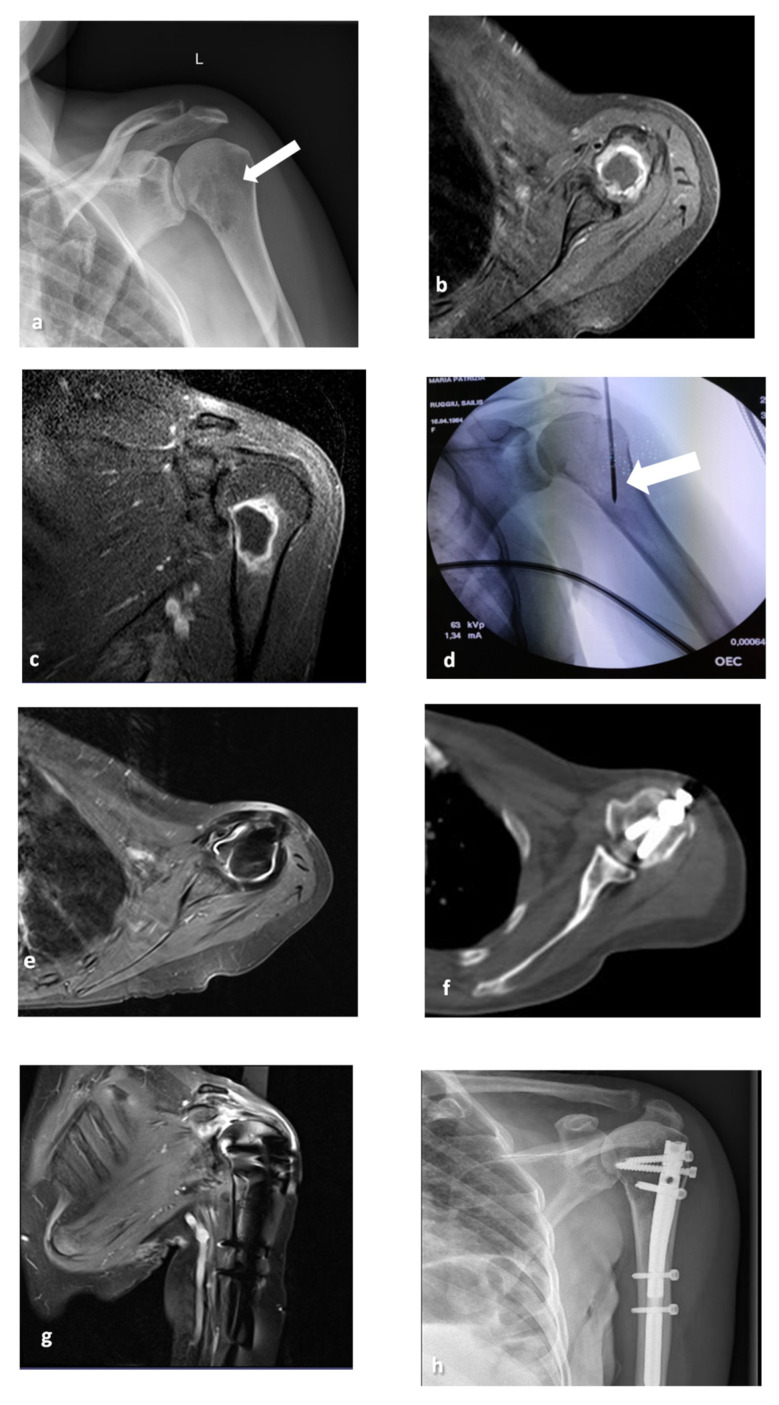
A 56-year-old female patient with a left humerus metastasis from breast cancer (a–d). Coronal X-ray shows a lytic lesion in the superior portion of the humerus (arrow). Axial and coronal T1-weighted MR scans with fat suppression after gadolinium infusion (b,c) show the lytic lesion surrounded by a rim of enhancement. The coronal C-Arm image (d) shows the MW antenna positioned in the lesion (arrow). After 1 year of follow-up, axial and coronal MRI (e–g), axial CT (f) and X-ray (h) show the presence of the endoprosthetic replacement and no local relapse in the treated site.
